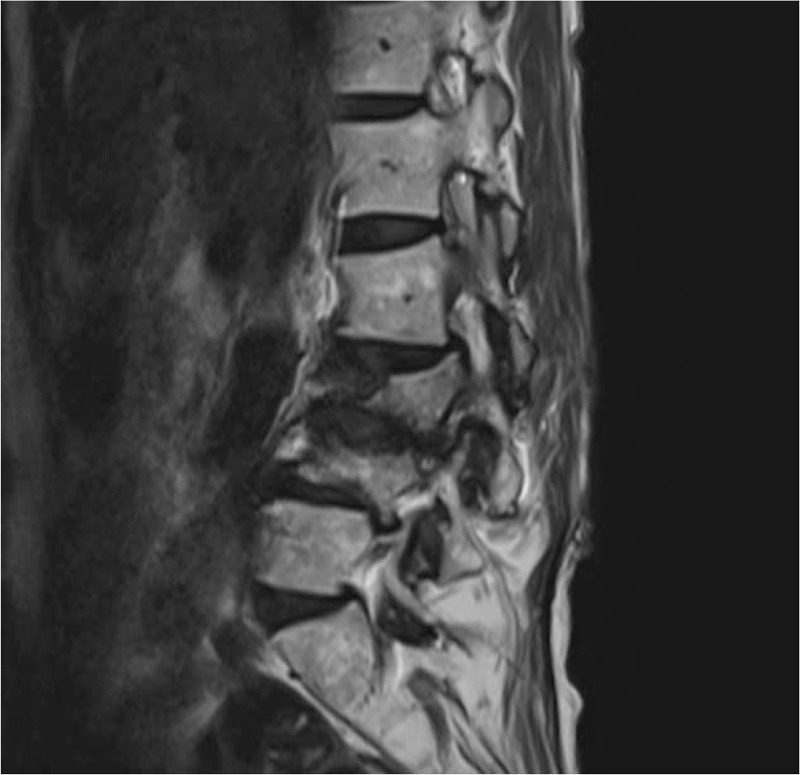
Sagittal image on MRI shows L3 compression fracture and L3 nerve root compression. The patient underwent a MEL for foraminal encroachment.
